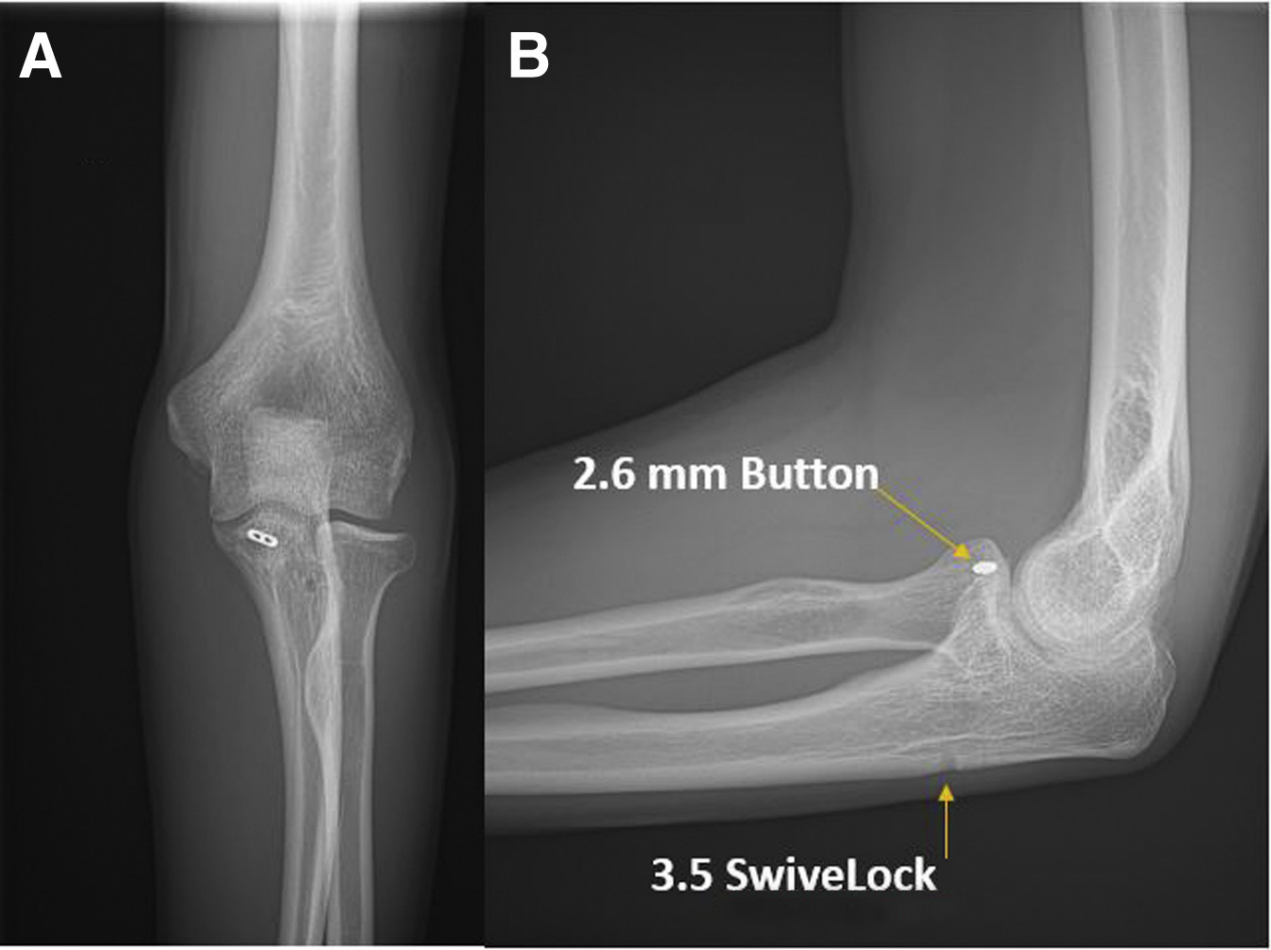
(A) Left elbow radiograph, anteroposterior view, demonstrates final fixation construct. (B) Left elbow radiograph, lateral view, demonstrating reduction of the coronoid fracture with suture button and knotless suture anchor and repair of the lateral collateral ligament complex. Note the posterior subluxation of the radial head has been corrected.
